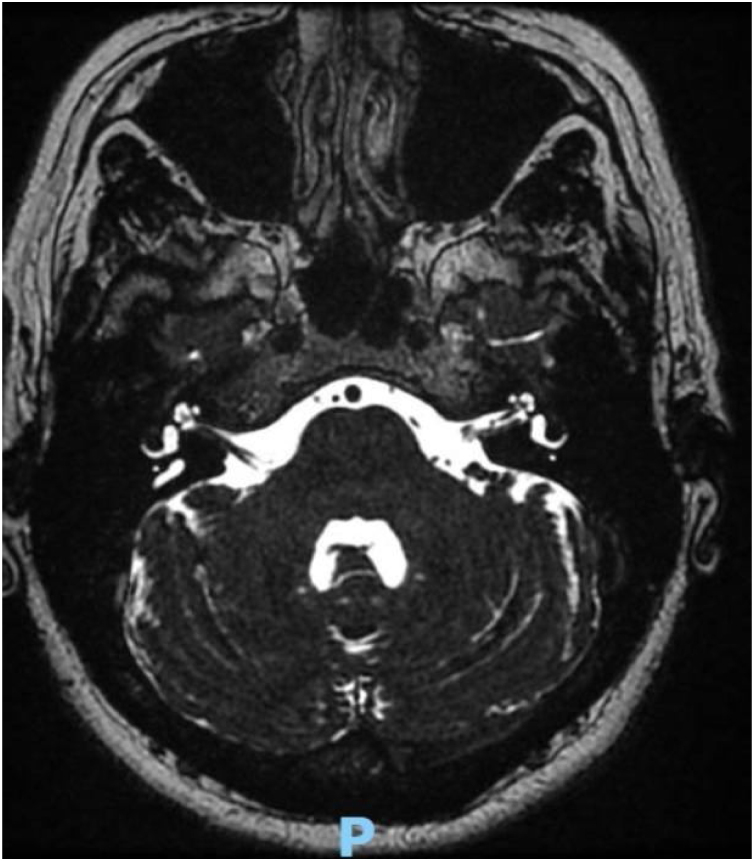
MRI scans: Malformation of the right inner ear, with dilation of the vestibular aqueduct and Alterations that may represent foci of gliosis and/or microangiopathy.
